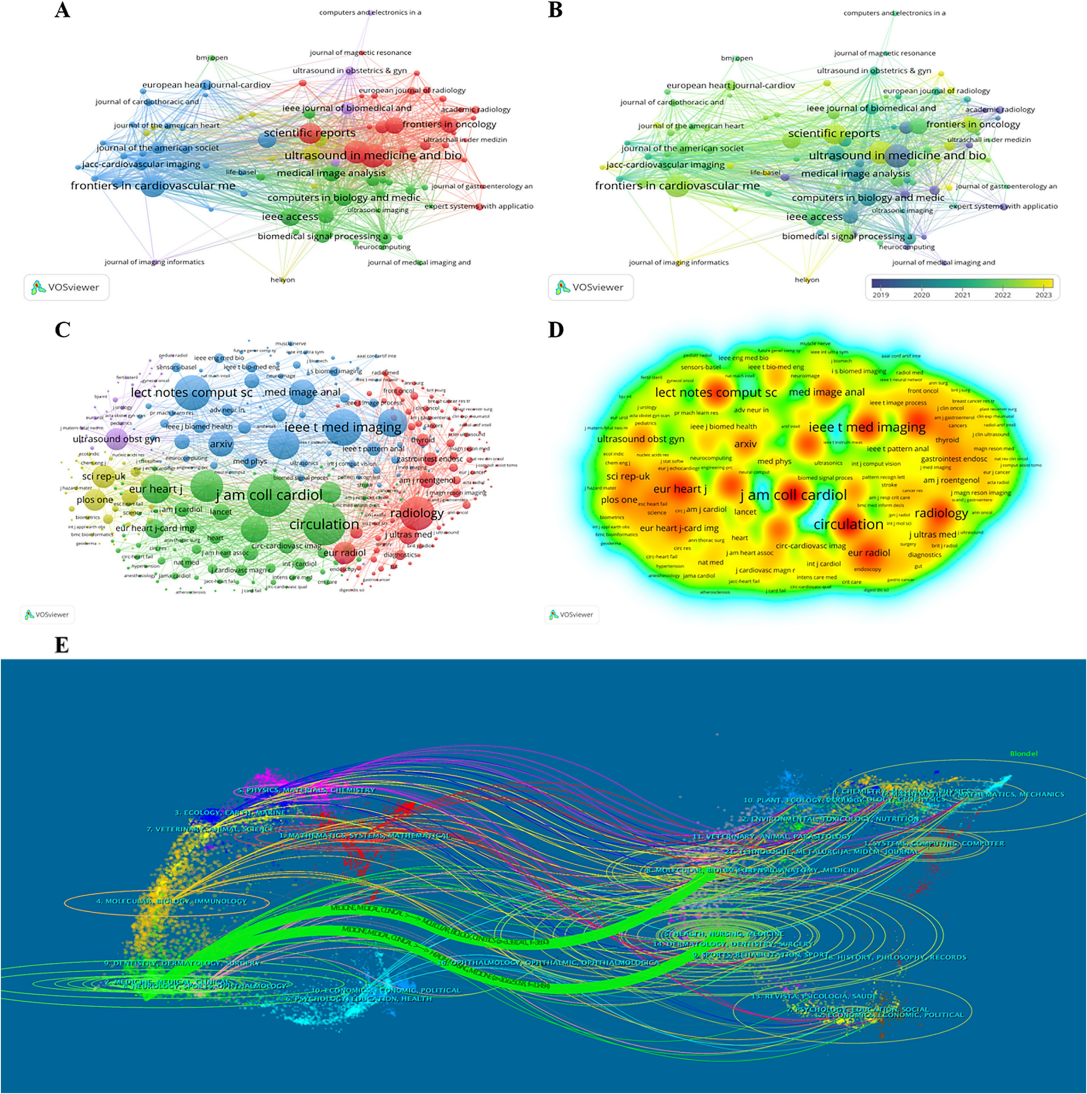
(A) Co-operation network between journals; (B) The time-overlay map of the cooperation network between journals; (C) Co-operation network between co-cited journals; (D) VOSviewer density visualization of the co-cited journals; (E) Dual-map overlay of journals related to the application of artificial intelligence in echocardiography.
